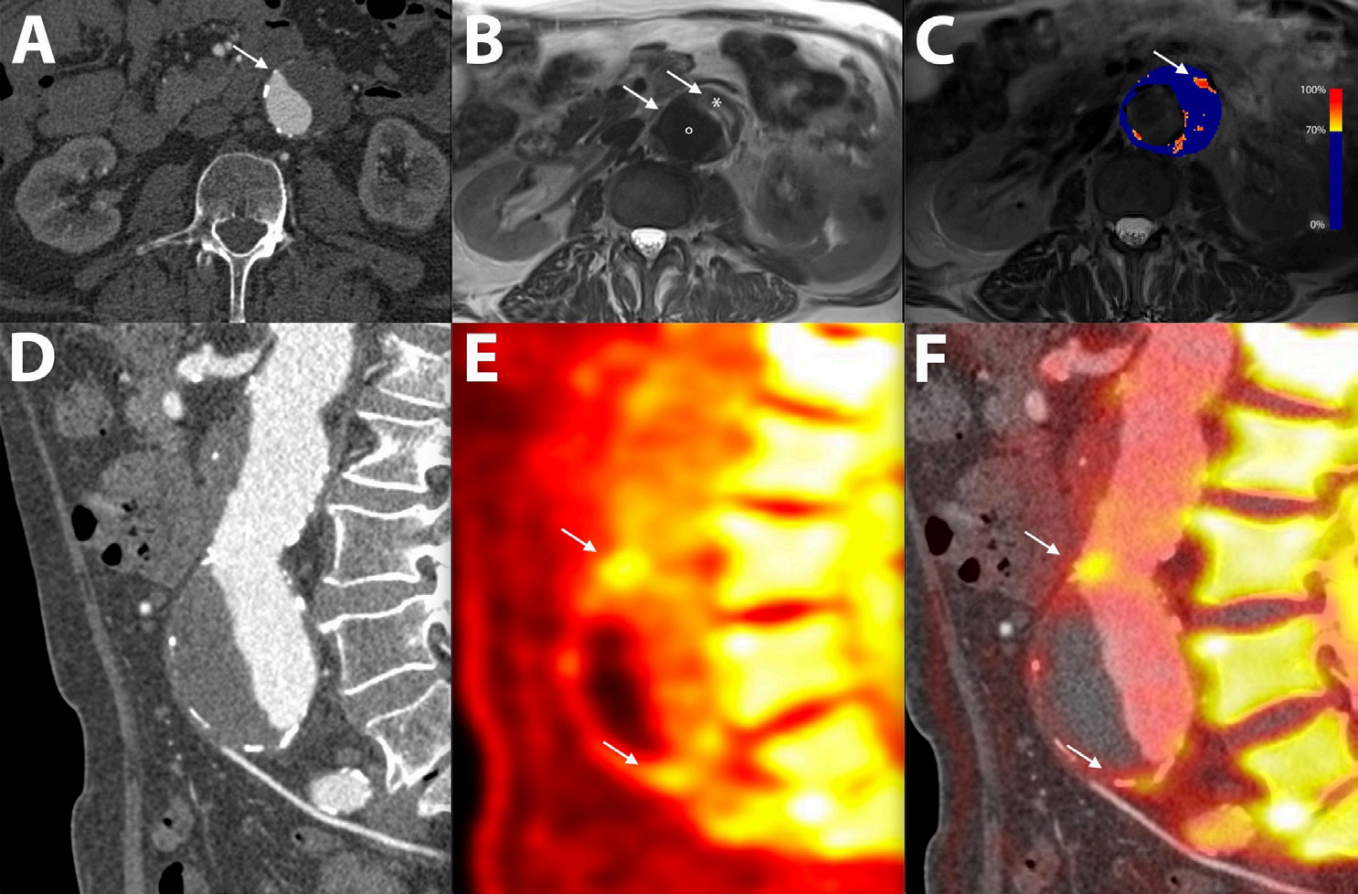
CT, MRI and PET in a patient with a juxtarenal abdominal aortic aneurysm. (A) Transverse view of the aneurysm as seen on CT shows a dilated aorta with thrombus. (B) *T*_2_ weighted MRI of the same aorta differentiates between the lumen (•), thrombus (*) and adjacent structures. (C) A parametric map of the difference in *T*_2_* MRI intensity before and after the administration of ultrasmall particles of iron oxide uptake shows high focal uptake in the anterior wall of the aneurysm (arrow). (D) The sagittal CT view delineates the morphology of the aneurysm. (E) ^18^F-Sodium fluoride PET shows uptake within the anterior aortic wall (arrows) detects areas of greatest vascular injury. (F) Superimposing PET over the CT confirms high ^18^F-Sodium fluoride uptake at the aneurysm neck and near the bifurcation (arrows). PET,positron emission tomography
